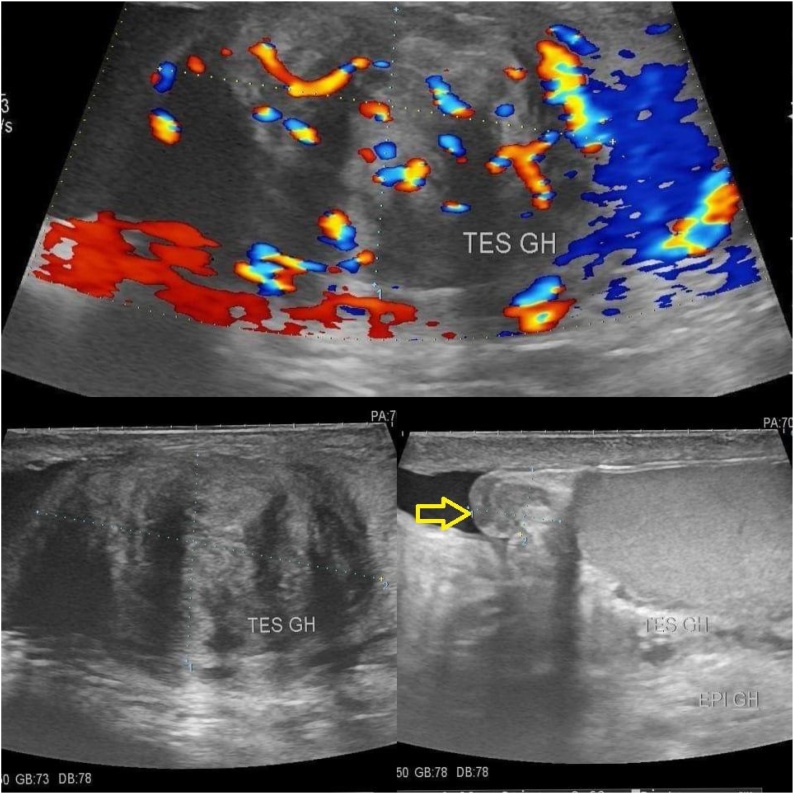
Ultrasound of the left testicle revealing a well circumscribed mass measuring 35 × 20 mm located in the upper pole.
